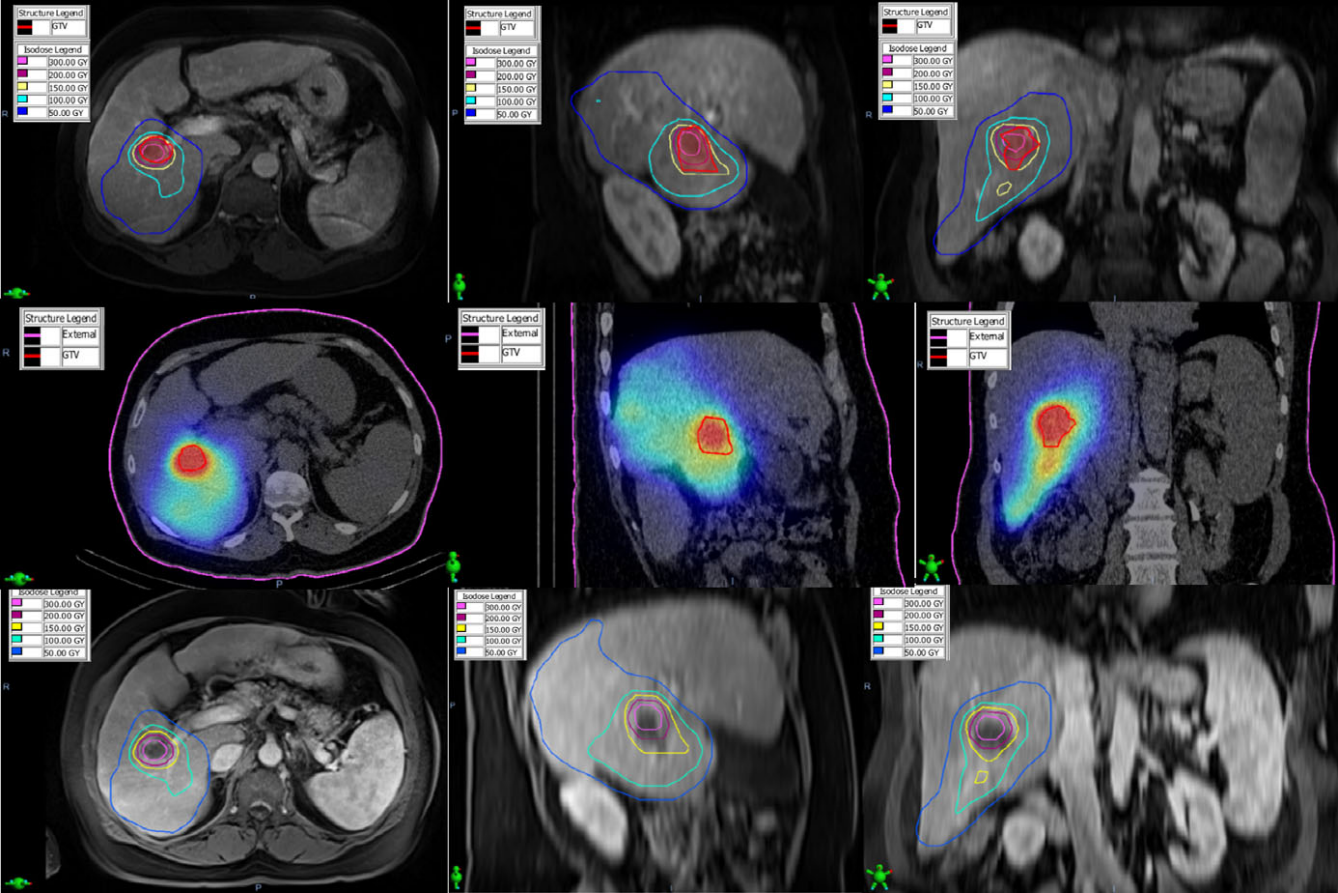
The deformably registered pre‐treatment diagnostic MR (top), post‐treatment SPECT/CT (middle), and the 5‐week follow‐up MR (bottom) for Case #1. Notice the GTV (red outline) is well covered by the prescription (150 Gy) isodose volume in the pretreatment MR and post‐treatment SPECT/CT which corresponds to a necrotic region in the follow‐up MR.
